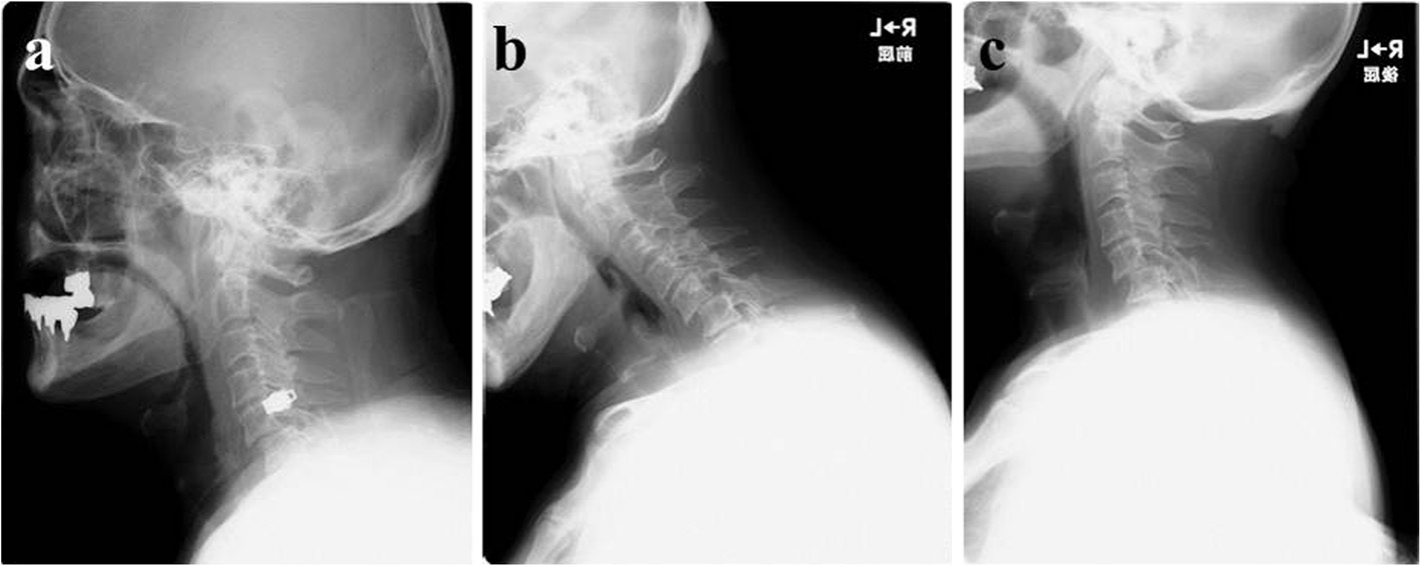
Plain X-ray film images showing slight spondylolisthesis of C5 immediately after a traffic accident. (a) The extent of the spondylolisthesis increased over the following eight weeks. (b) Flexion position; (c) extension position.
